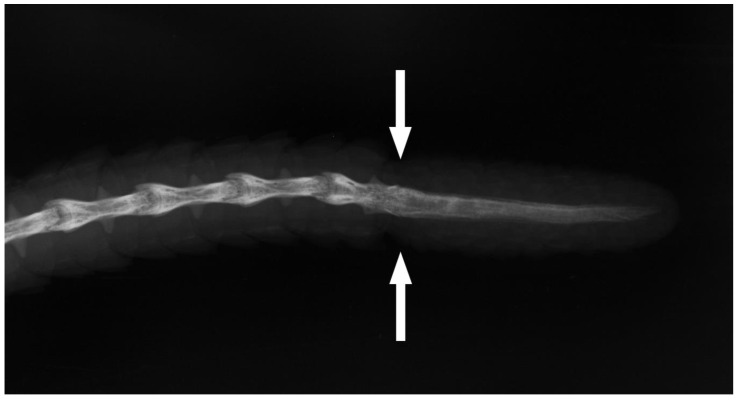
X-ray photograph of *Paralaudakia caucasia* ZISP 19718 (Turkmenistan) with the pseudoautotomy in the distal part of the tail. The partial ablation at the site of the autotomy and the formation of the cartilaginous tube are shown.
